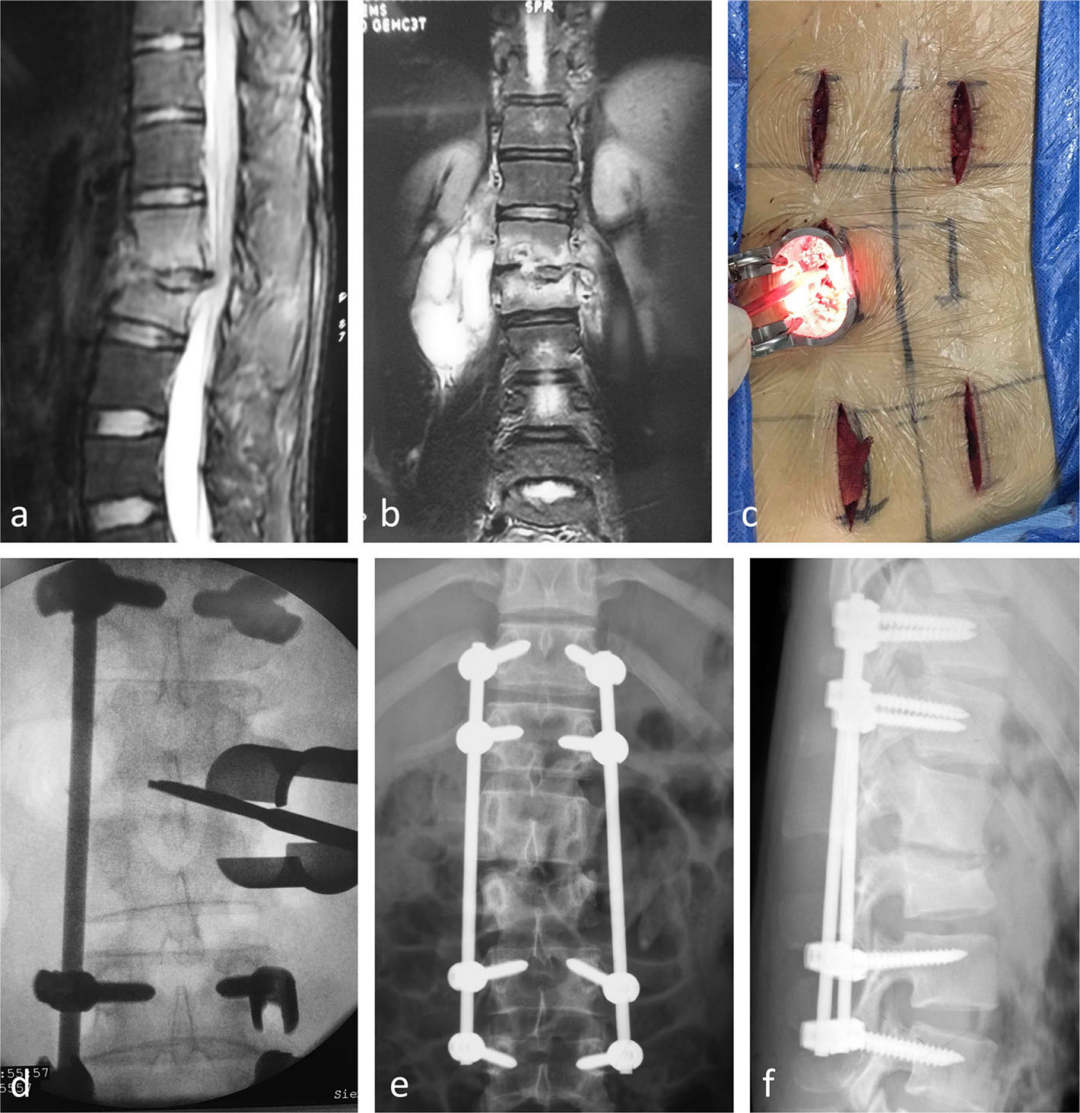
A case of a 16-year-old male suffered from back pain with L1/2 tuberculosis. a Preoperative sagittal MRI showed tuberculosis which involves the intervertebral disc, leading to the kyphosis. b Preoperative coronal MRI showed psoas abscess and destruction of intervertebral disc. c Five 3 cm-long incisions were carried out, four of them for the pedicle screws insertion, and the fifth for debriding the TB lesion. d Expandable tubular retractor was docked over the right side facet joint, and the TB lesion was cleared through the far lateral approach. e, f Postoperative anteroposterior and lateral radiographs after long segmental fixation showed acceptable spinal alignment
